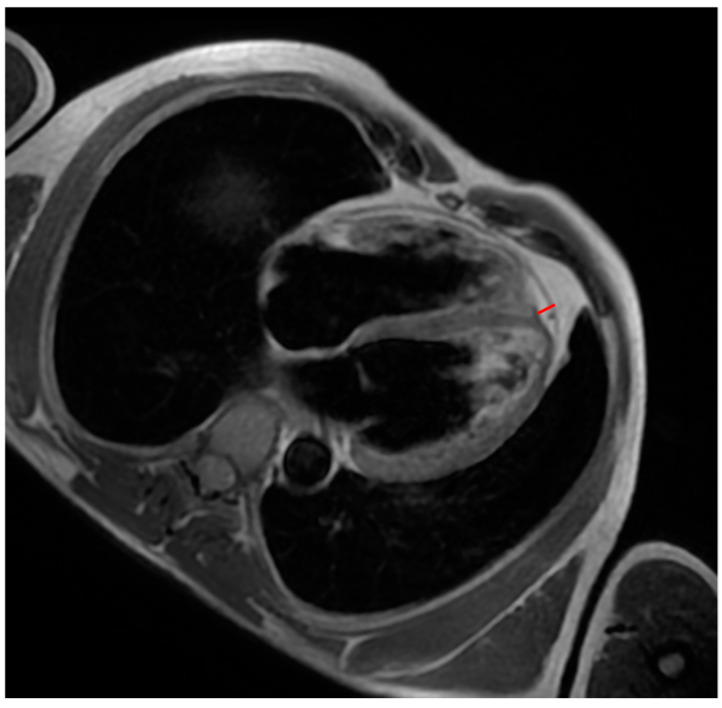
A patient with ACM. Black-blood T1-weighted 4-chamber image shows an example of measuring interventricular groove EAT (red line).
